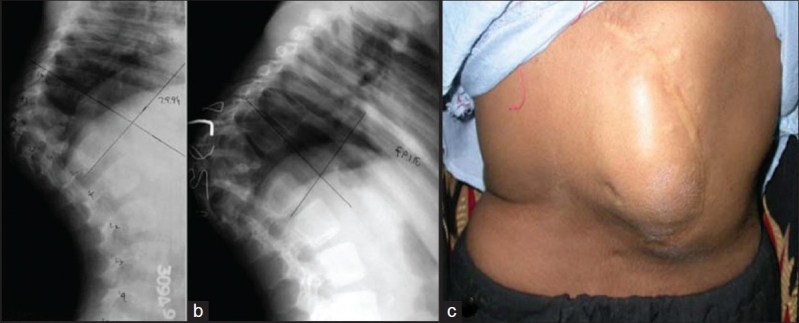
Lateral X-ray of a dorsolumbar spine (a) shows a severe kyphosis secondary to childhood tuberculosis spine with complete destruction of D9–11. This kyphosis is expected to grow with growth. Lateral X-ray of the same patients (b) 2½ years later shows progression of kyphosis in spite of successful posterior spinal fusion. Clinical photograph (c) of the same patient shows deformity and scar of posterior fusion
